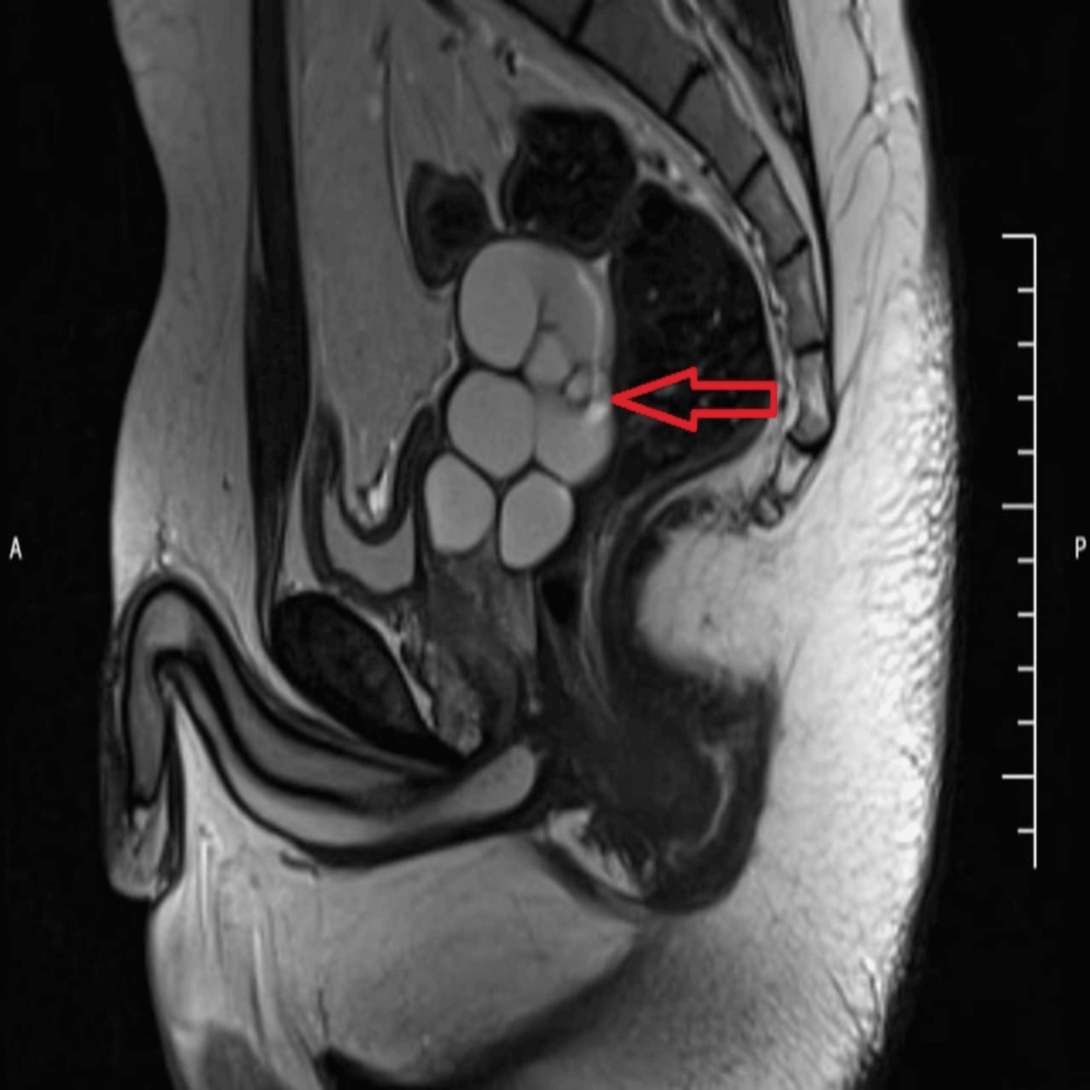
Sagittal T2-weighted MRI pelvis image reveals a hyperintense, tubular seminal vesicle cyst with incomplete septations adjacent to the prostate in the retrovesical space (arrow). MRI, Magnetic resonance imaging
